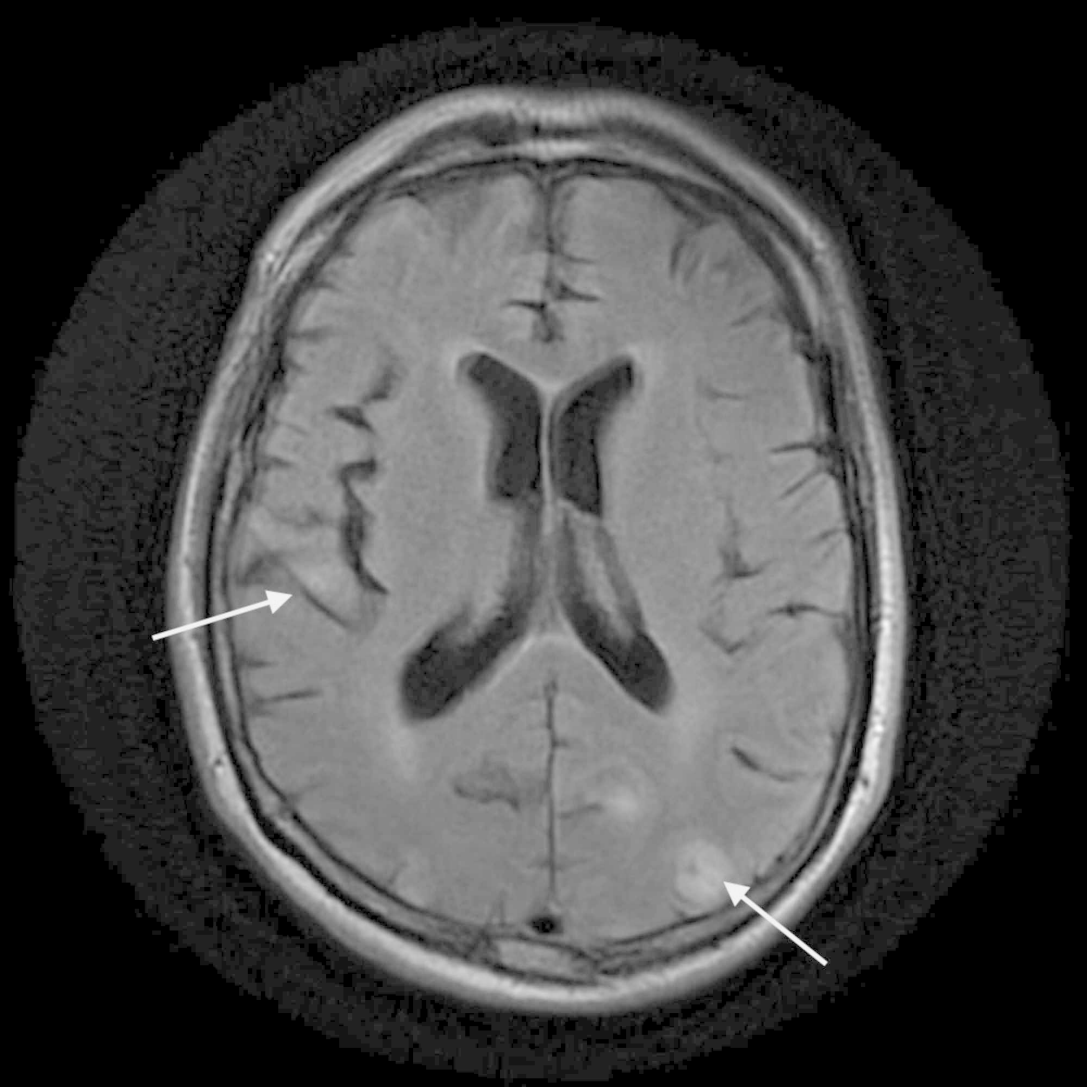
T2 FLAIR MRI of the brain with and without contrast. T2 fluid attenuated inversion recovery (FLAIR) MRI of the brain showed new multifocal areas of abnormal FLAIR hyperintensity signals in the supratentorial cortices and subcortical white matter (arrows).
